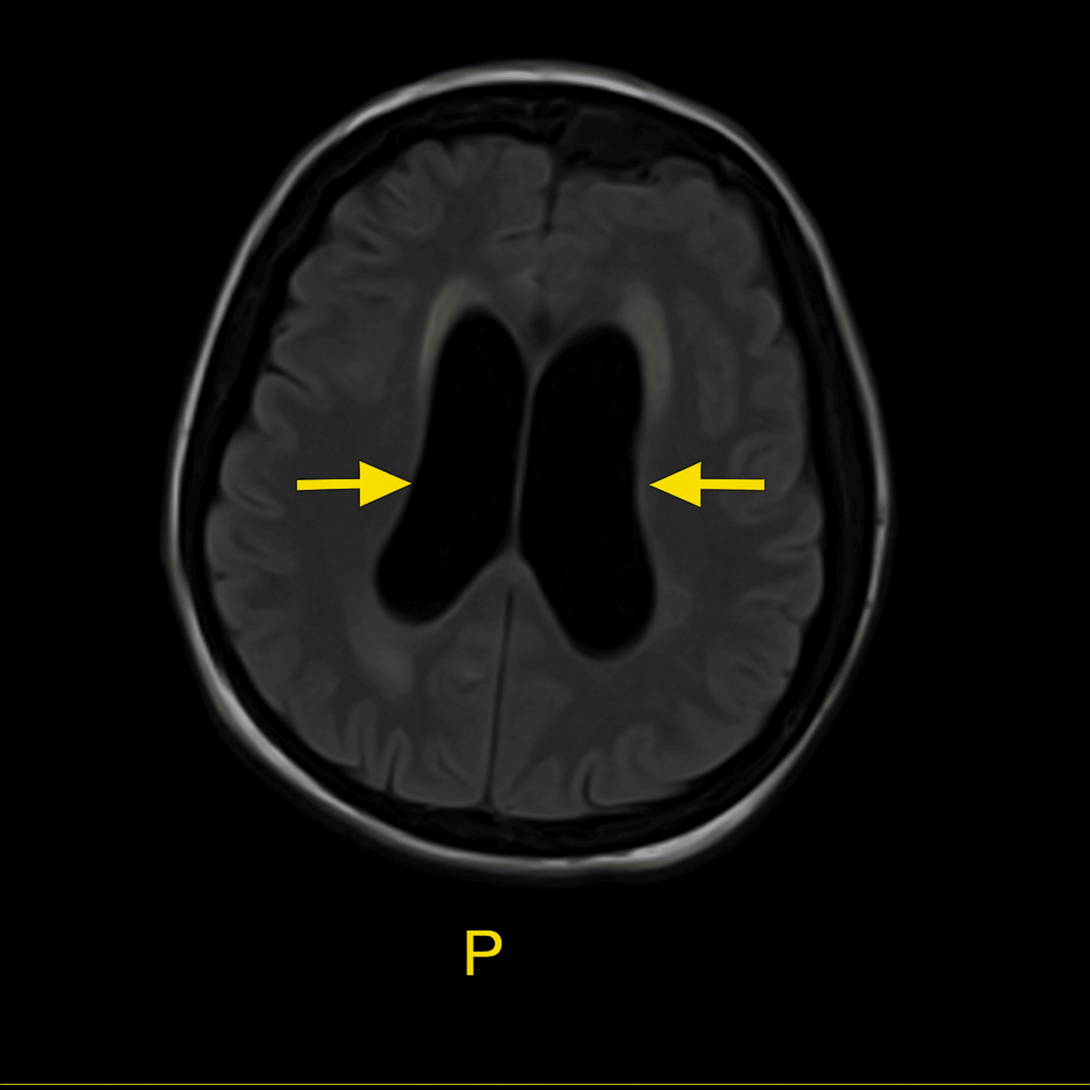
Axial T2-weighted FLAIR MRI demonstrating dilated lateral ventricles (arrows) consistent with ventriculomegaly FLAIR - fluid-attenuated inversion recovery
